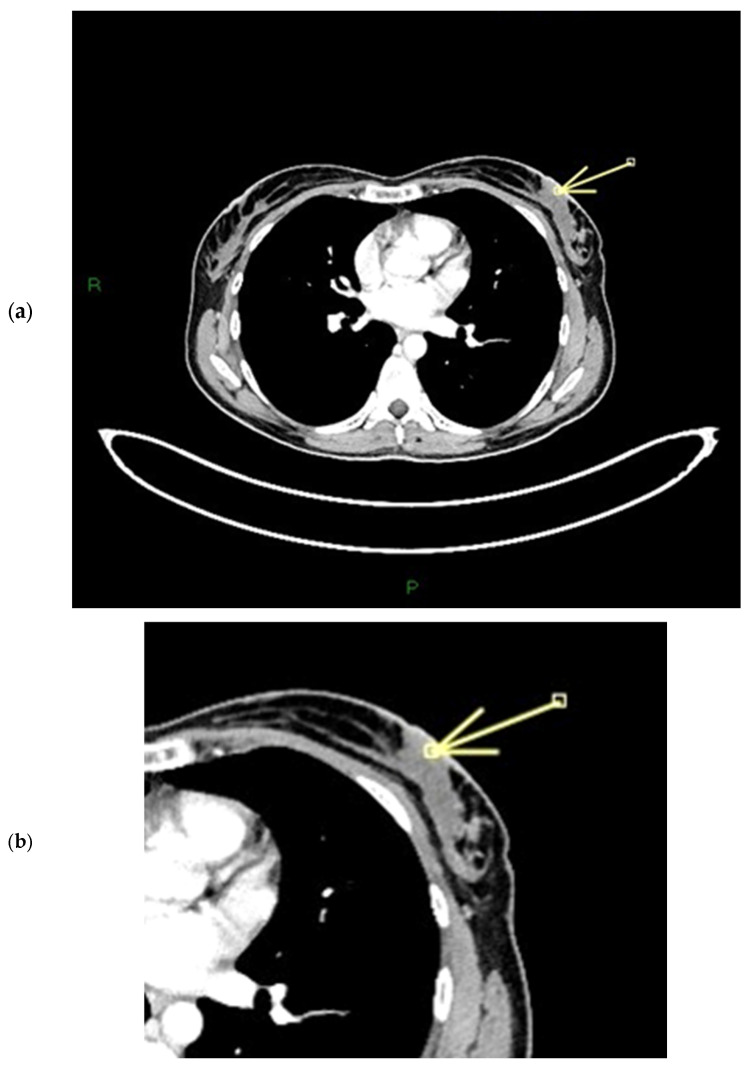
(a,b) Patient’s name M.E. The ce-CT scan shows enhancement of the base of the left nipple alone (no body; arrow). Morphology of the nipple is everted.
